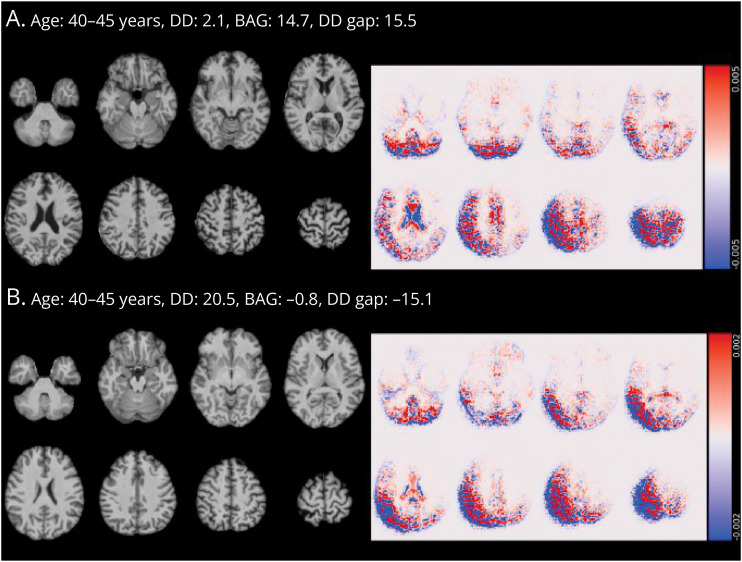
Guided Backpropagation Analysis to Interrogate Brain Regions Influencing the Model for the Prediction of Disease Duration Lightbox view of selected slices from the quasi-raw T1w volumes (on the left) and corresponding guided backpropagation–derived saliency maps (on the right) of 2 representative PwMS exhibiting extremely positive (A) or negative (B) values of the DD gap. For saliency maps, both positive (positively correlated with the output, in red) and negative (negatively correlated with the outcome, in blue) magnitudes are shown. In both cases, the model focuses mostly on regions that seem to be related to (the widening of) the CSF spaces. BAG = brain-age gap; DD = disease duration; PwMS = patients with multiple sclerosis; T1w = T1-weighted.
